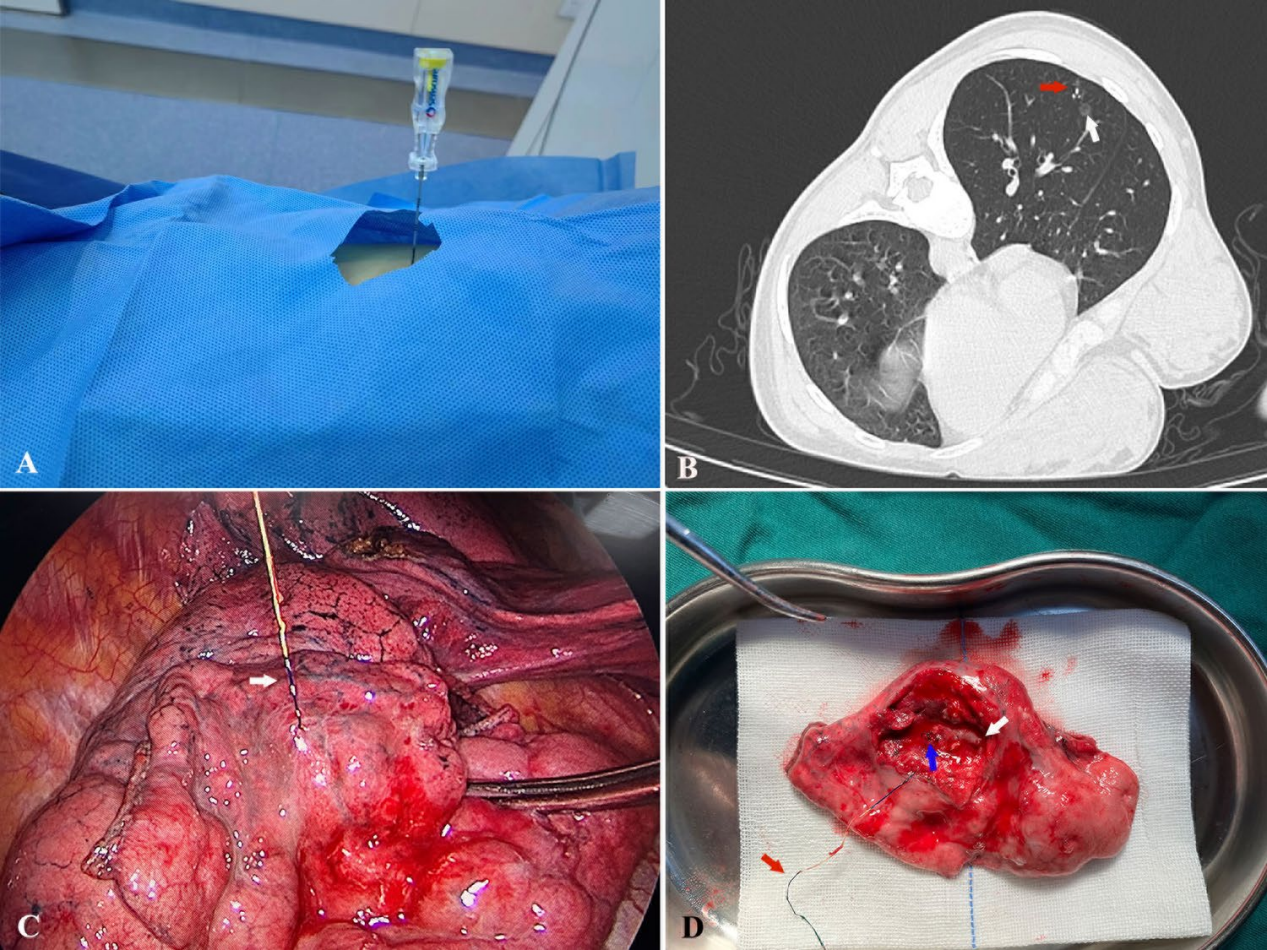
The main process of localization for patients with SPNs. (A) The coaxial needle was inserted through the chest wall based on the location of the pulmonary nodule after CT scan. (B) CT image shows the pulmonary nodule (white arrow) and the claw (red arrow). (C) The tri-colored suture (white arrow) was displayed during the first exploration by VATS. (D) The claw (blue arrow) and the tri-colored suture (red arrow), and the localized pulmonary nodule (white arrow) were displayed after wedge resection.
